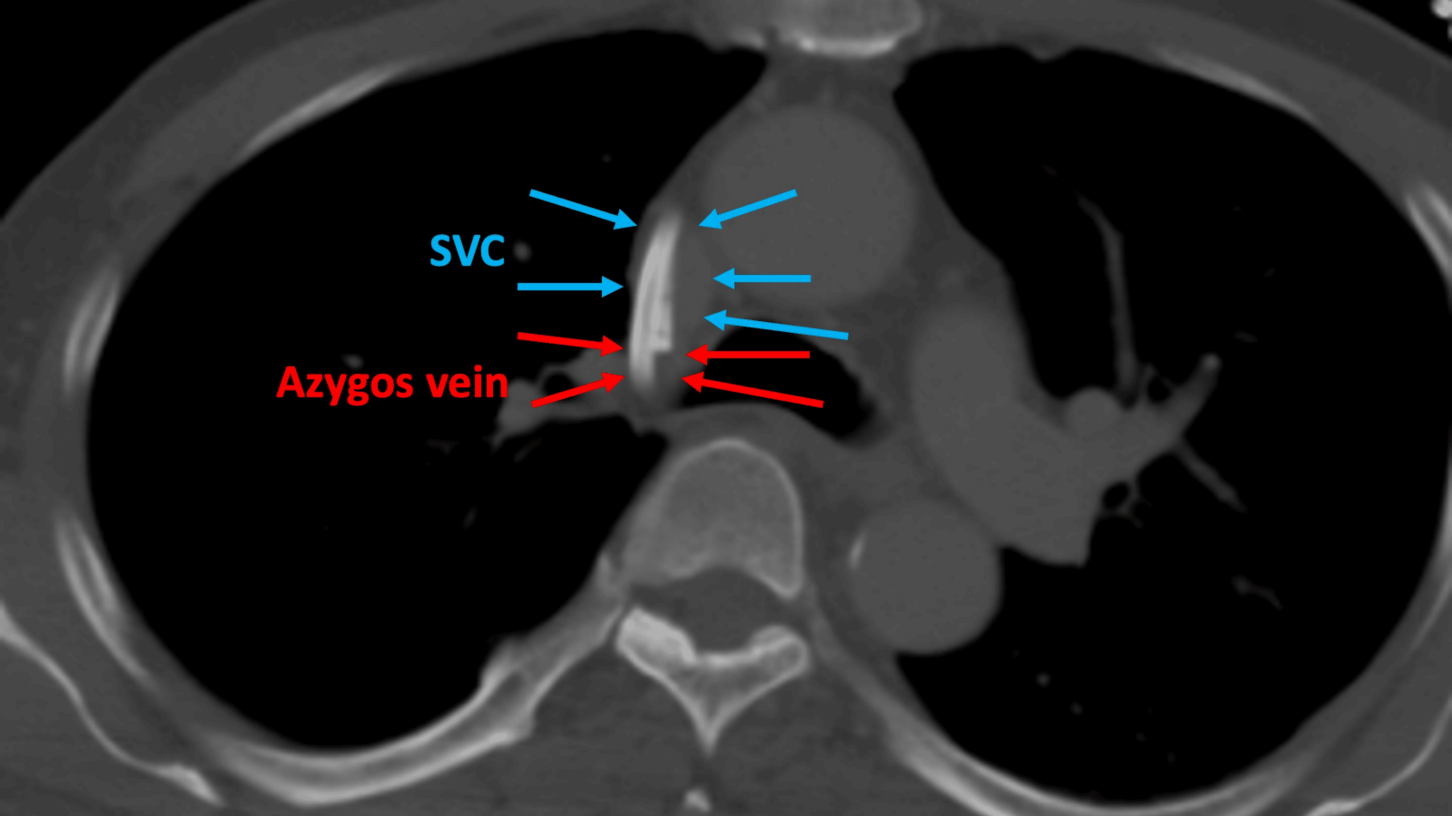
Contrast CT thorax axial slice Confirmed malposition of both catheter tips into the azygos vein (red arrows). Blue arrows denote SVC. CT: computed tomography, SVC: malposition of central venous catheter
